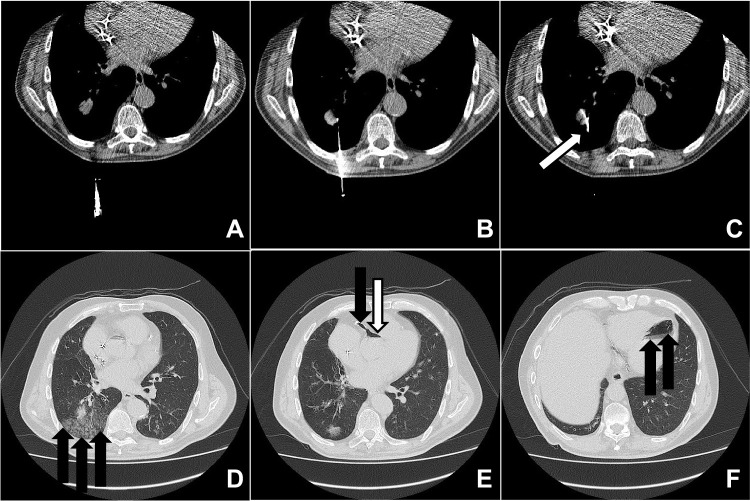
CT scans in sequence obtained during the procedure (A, B, and C) and sequential slices of scan performed after the procedure (D, E, and F). A First image in the sequence showing the lesion (light gray mass) within the right lung lobe. B  Image in the sequence showing needle being inserted through the skin of the back. C Image showing the 17-gauge BioPince-type needle within the lesion (indicated by the white arrow). D Image showing hemorrhage along the posterior aspect of the right lung (black arrows). E Image showing air within the aorta (white arrow) and right coronary artery (black arrow). F Image showing air within the left ventricle (black arrows)
